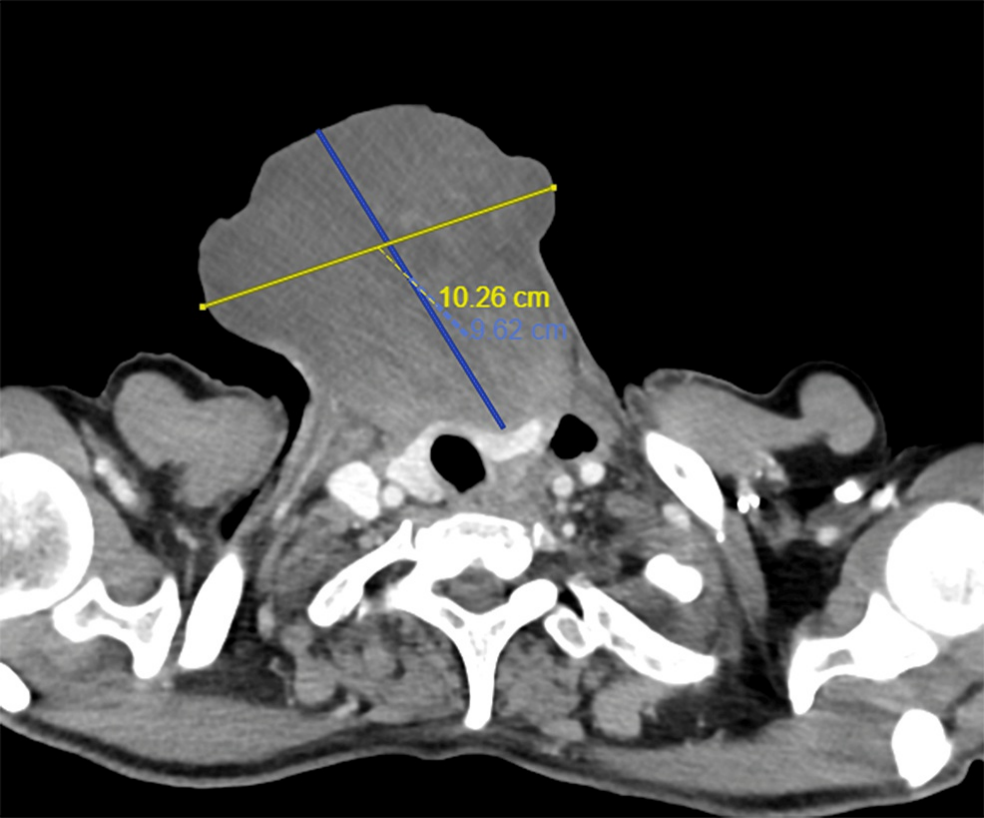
An axial section of the sarcomatoid carcinoma of esophagus, as seen on CECT imaging. CECT: contrast-enhanced computed tomography
